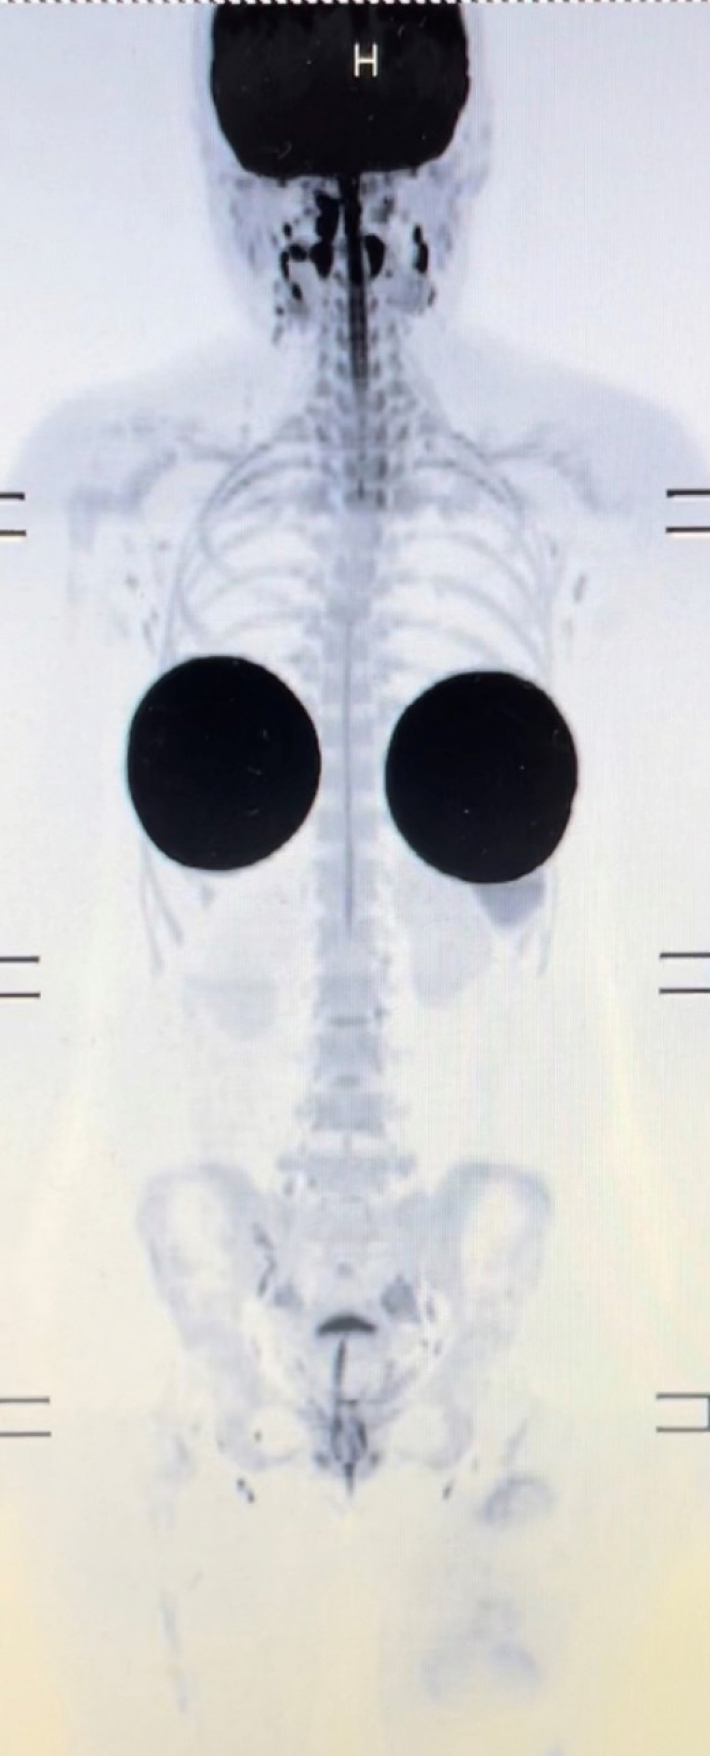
Representative image of whole-body magnetic resonance imaging surveillance
